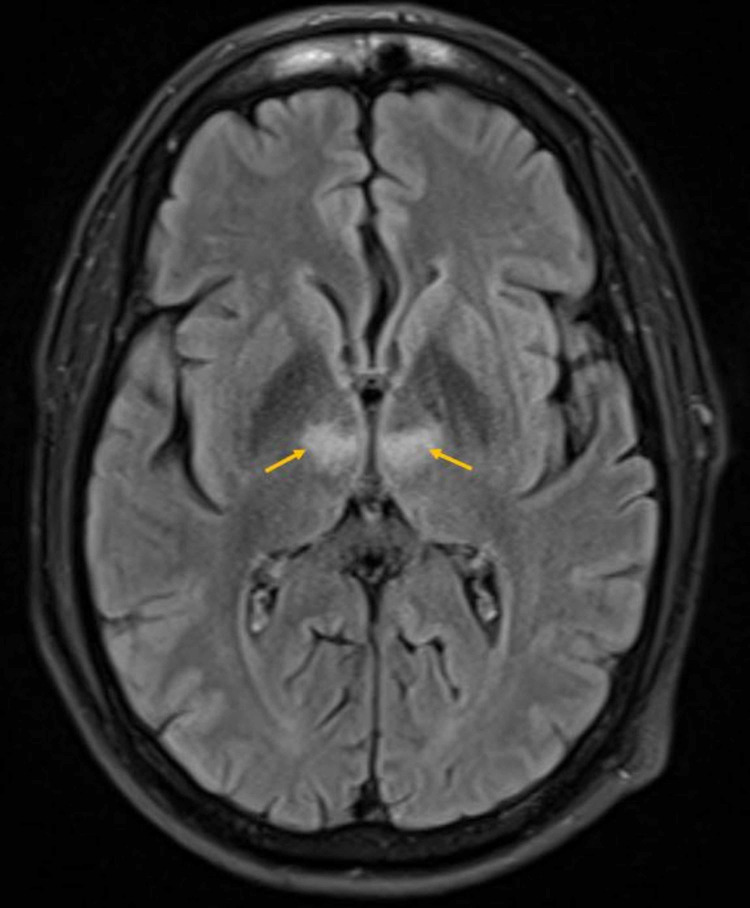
Brain MR fluid-attenuated inversion recovery (FLAIR) axial image. Bilateral anterior thalamic high signal intensity corresponding to the areas of diffusion restriction.
